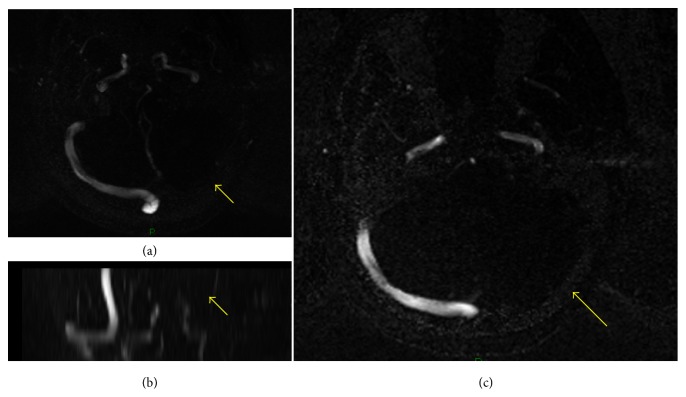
Loss of flow signal intensity in left lateral sinus which is showing heterogeneous appearance on FLAIR and T2-weighted images suggesting left lateral sinus thrombosis with venous infarction.
